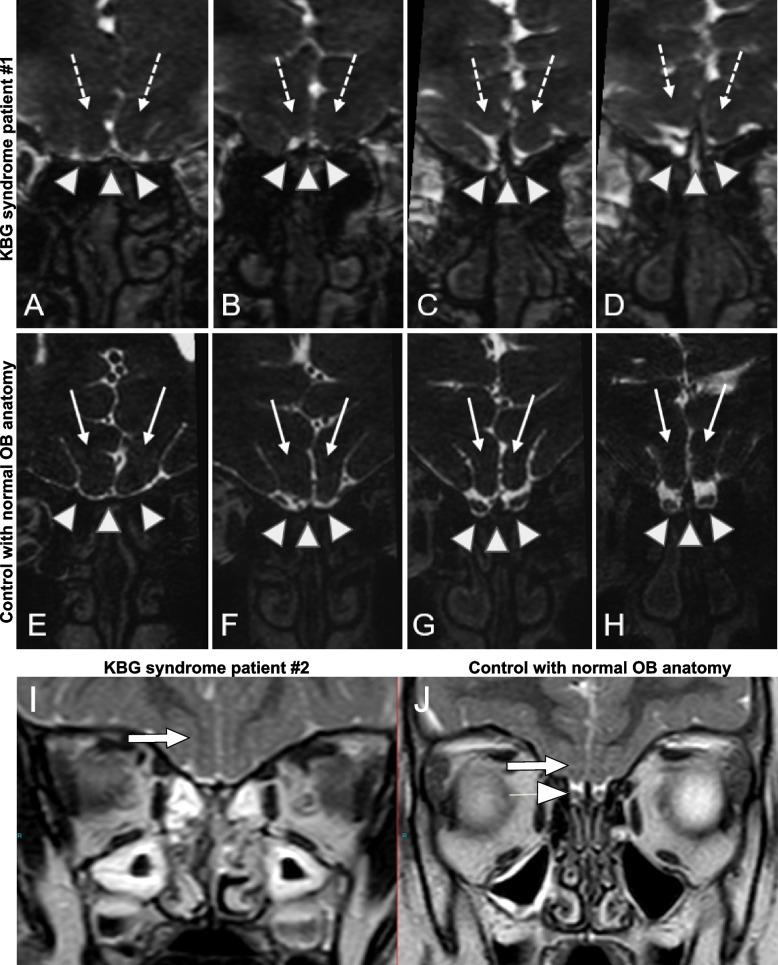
OB hypoplasia in KBG syndrome patients. Coronal multiplanar reformatting three-dimensional high resolution heavy T2-weighted images of the naso-ethmoidal region. A–D Patient #1; MRI at 14 years of age. E–H Normal control anatomy. The olfactory bulb is not clearly visible in KBG syndrome patient as compared to the normal subject (arrowheads). In addition, the olfactory grooves are severely hypoplastic and the straight gyri (dotted arrows in A–D) are dysmorphic with less prominent and organized adjacent sulci with respect to the normal anatomy (arrow in E–H). I Patient #2; MRI at 3 years of age. J Normal control anatomy. No olfactory bulbs are seen in the KBG syndrome patient and consequently there are no olfactory grooves. The olfactory sulci are visible (arrow). Olfactory bulbs (arrowhead) are visible within well-formed olfactory grooves of the control. The olfactory sulci (arrow) can also be seen
